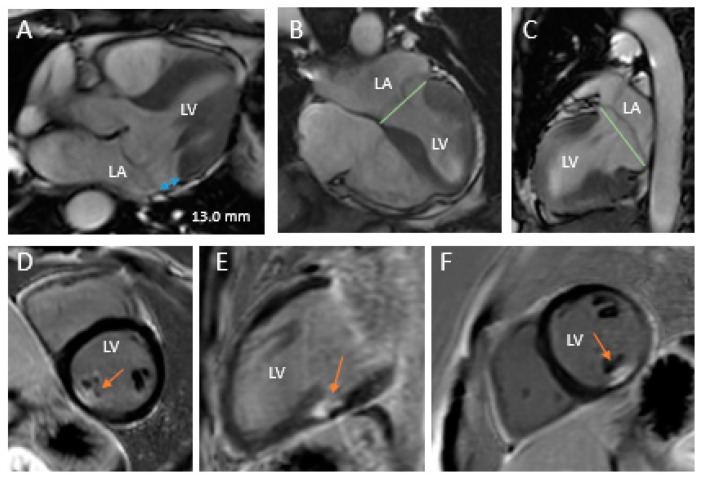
CMR examples of patients with Barlow’s disease. CMR images of two patients with Barlow’s disease. Panels (A–C) represent Patient 1 with Barlow’s disease, showing mitral annular disjunction (blue arrow) and a clear bileaflet prolapse. Panels (D–F) represent Patient 2 with late gadolinium enhancement at the level of the papillary muscle (orange arrow). CMR = cardiac magnetic resonance imaging, LA = left atrium and LV = left ventricle.
